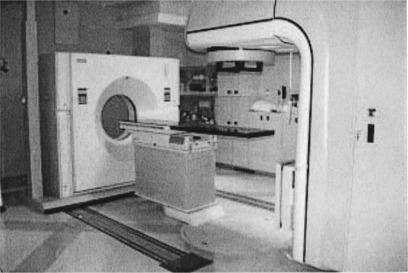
(Color) CT scanner in the same room as a linear accelerator, sharing a single patient couch.
